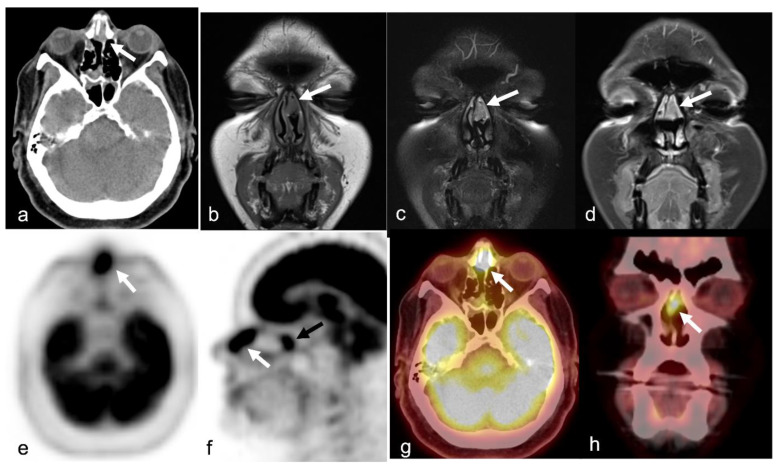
Sinonasal squamous cell carcinoma. A 49-year-old female with a history of nasal obstruction and occasional nose bleeds for a few months. The axial unenhanced CT image (a) demonstrates a left anterior nasal mass (white arrow). On corresponding coronal MRI images (b–d), the mass that is isointense relative to muscles on T1w (b) is heterogeneously hyperintense relative to muscles on fat-saturated T2w images (c), showing homogeneous enhancement on postcontrast T1w fat-saturated (d) images (white arrow). Attenuation-corrected axial (e), sagittal (f) PET, and axial (g) and coronal (h) fused PET/CT images demonstrate high FDG metabolism of the lesion (white arrow). On image (f), a surgically proven second squamous cell carcinoma focus on the left posterior nasal cavity is seen (black arrow).
